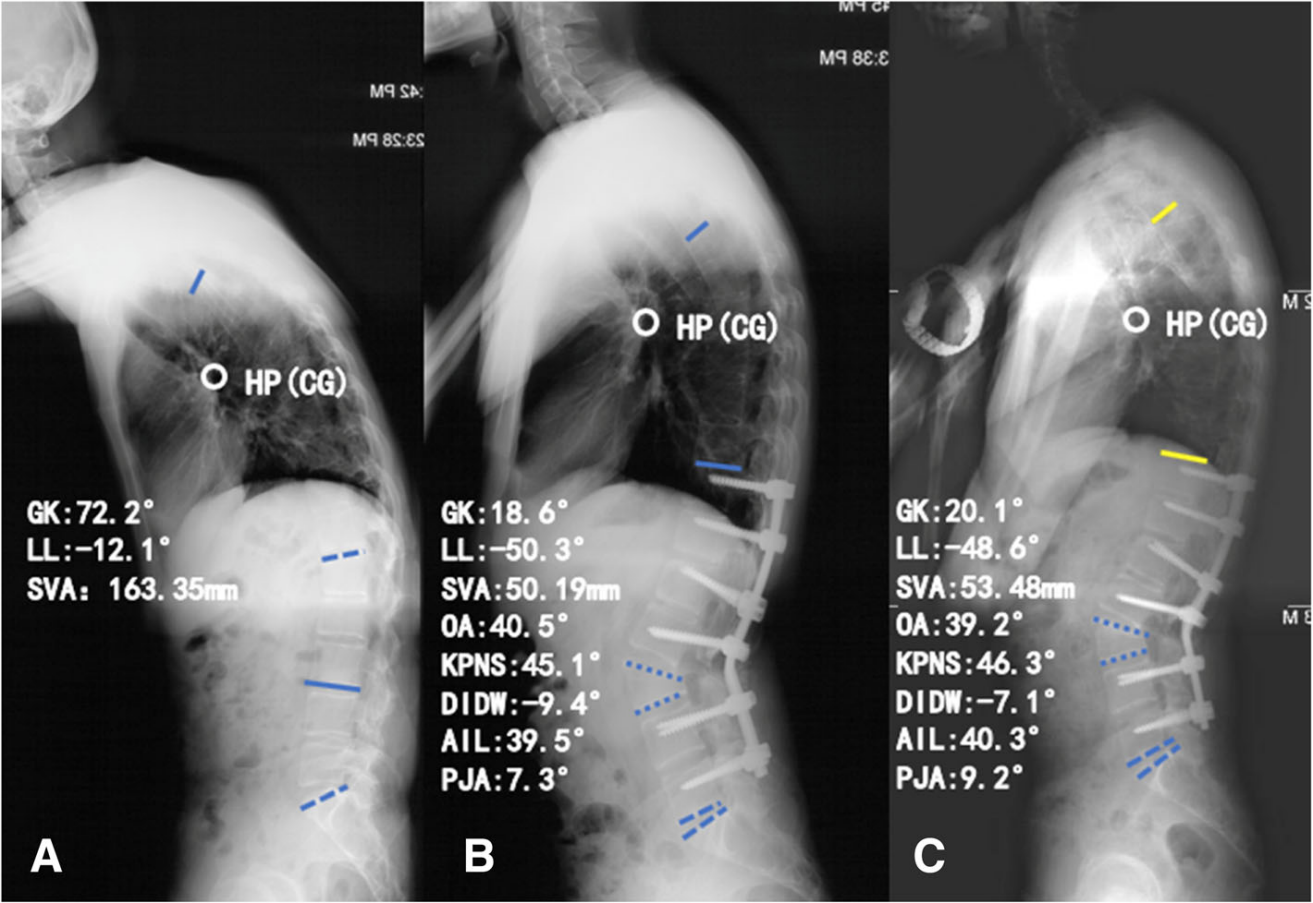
A 37-year-old male patient with thoracolumbar kyphosis secondary to ankylosing spondylitis. a Preoperative standing lateral radiograph. b Immediately after surgery with pedicle subtraction osteotomy (PSO) technique. c Lateral radiograph taken 26 months after surgery. AIL angle of instrumented levels, DIDW the sum of distal non-fused intervertebral disc wedging, GK global kyphosis, KPNS kyphotic angle of proximal non-fused segment involved in the global kyphosis, LL lumbar lordosis, OA osteotomized vertebra angle, PJA proximal junctional angle, SVA sagittal vertical axis, HP hilus pulmonis, CG the center of gravity
